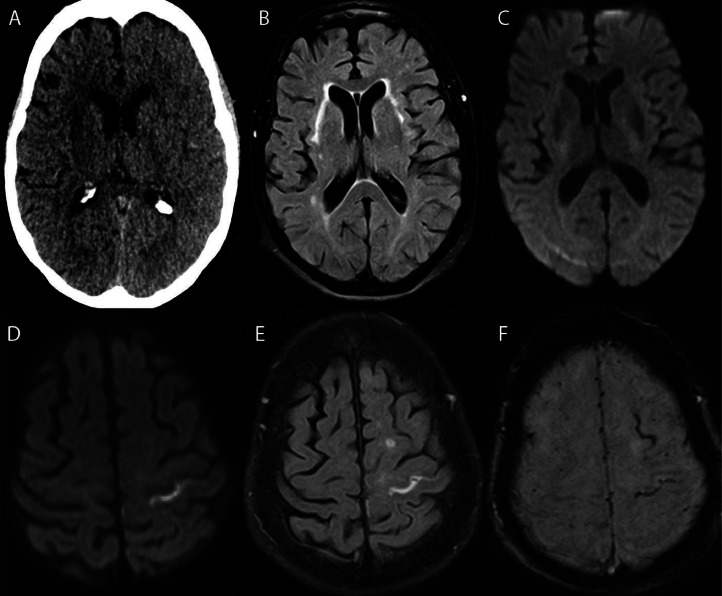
Imaging studies from Case 2. Non-contrast CT (A) performed 30 minutes after symptom onset demonstrates profound left hemispheric edema. 3.0 Tesla MRI performed 48 hours later demonstrates resolved edema on FLAIR images (B) and no acute infarction on DWI (C) in the previously affected regions; in the left central sulcus, DWI (D), FLAIR (E), and susceptibility-weighted imaging (F) demonstrate diffusion restriction, linear FLAIR non-suppression, and susceptibility artifact compatible with extravasated contrast or trace subarachnoid blood.
